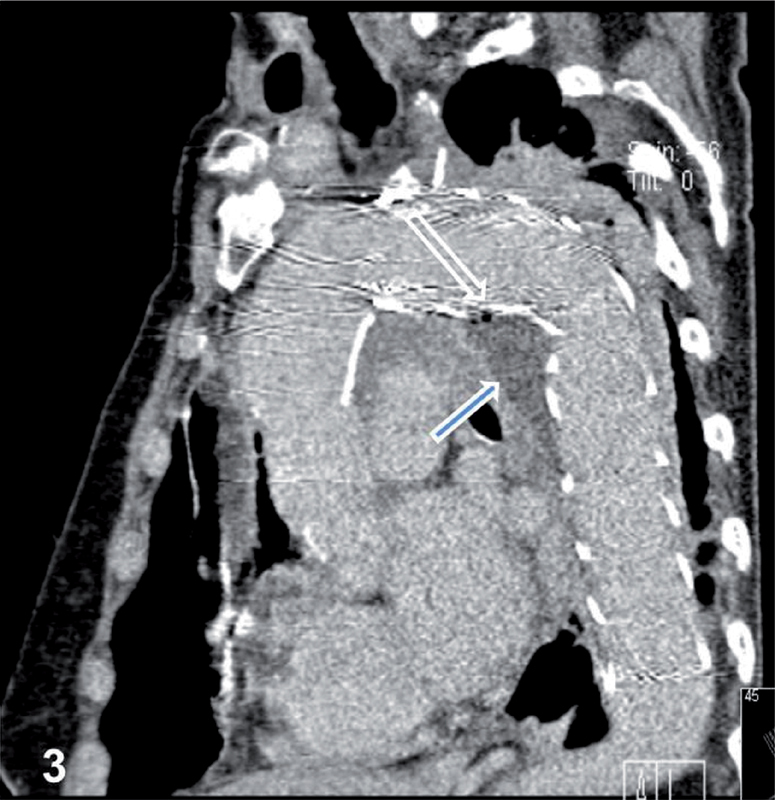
Computed tomography scan showing the stent graft surrounded by infected soft tissue (arrow) with air bubbles (outlined arrow) in the distal aortic arch and proximal descending thoracic aorta.
